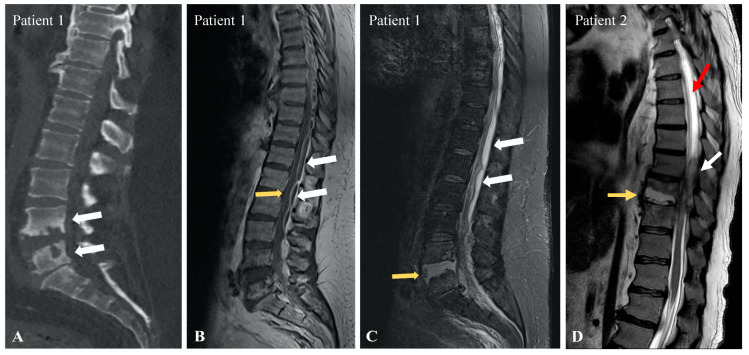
(A): Lumbar Computed Tomography showing L4 and L5 erosions (white arrows) due to L4-L5 spondylodiscitis. (B): Lumbar sagittal T1-weighted magnetic resonance imaging (MRI) showing multiple epidural abscesses (white arrows) with compressions of the spinal canal (yellow arrow). (C): Lumbar sagittal T2-weighted Short Inversion Time Inversion Recovery (STIR) MRI showing L4-L5 discitis (yellow arrow) with epidural abscesses (white arrows). (D): Thoraco-lumbar sagittal T2-weighted MRI showing T9–10 spondylodiscitis (yellow arrow), with epidural abscess (white arrow) and hypersignaling of the CSF above the compression (red arrow).
